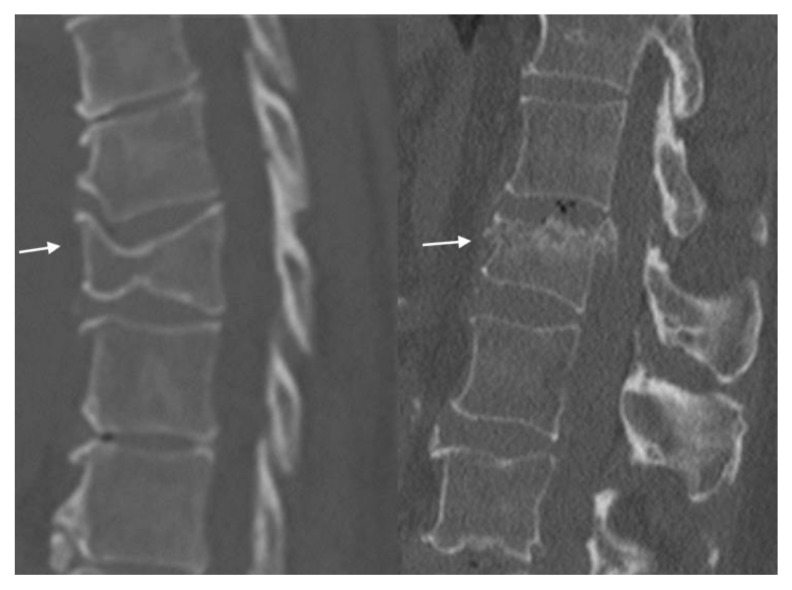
In this sagittal CT (left), the arrow shows a T8 vertebral body wedge compression fracture with moderate loss of vertebral height. In this sagittal CT (right), the arrow shows an acute to subacute burst compression fracture of the superior endplate of L1 with approximately 40% height loss. The bones are diffusely demineralized.
